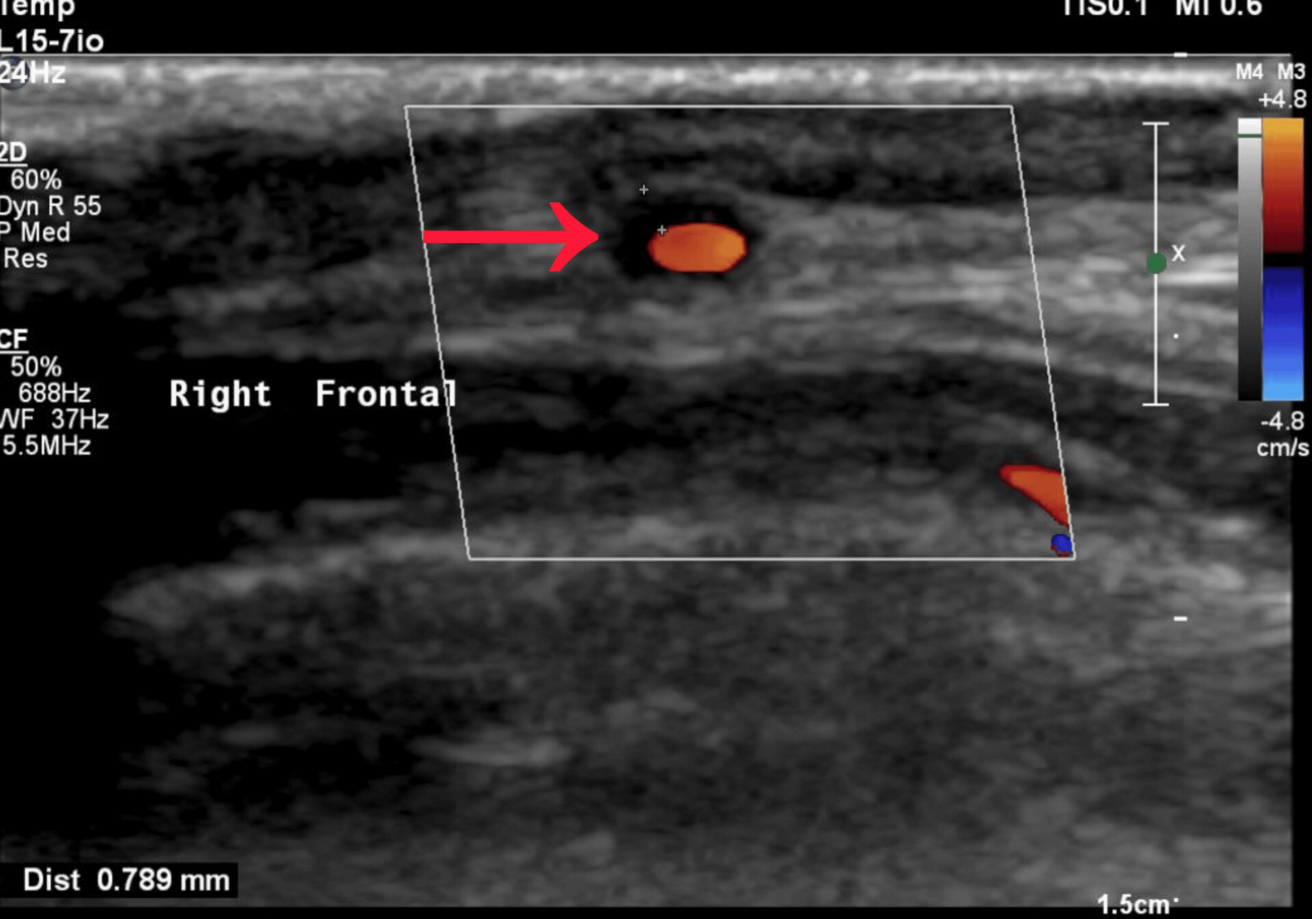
Ultrasound Doppler of temporal artery showing halo sign (dense vessel wall thickness with a narrow lumen).
